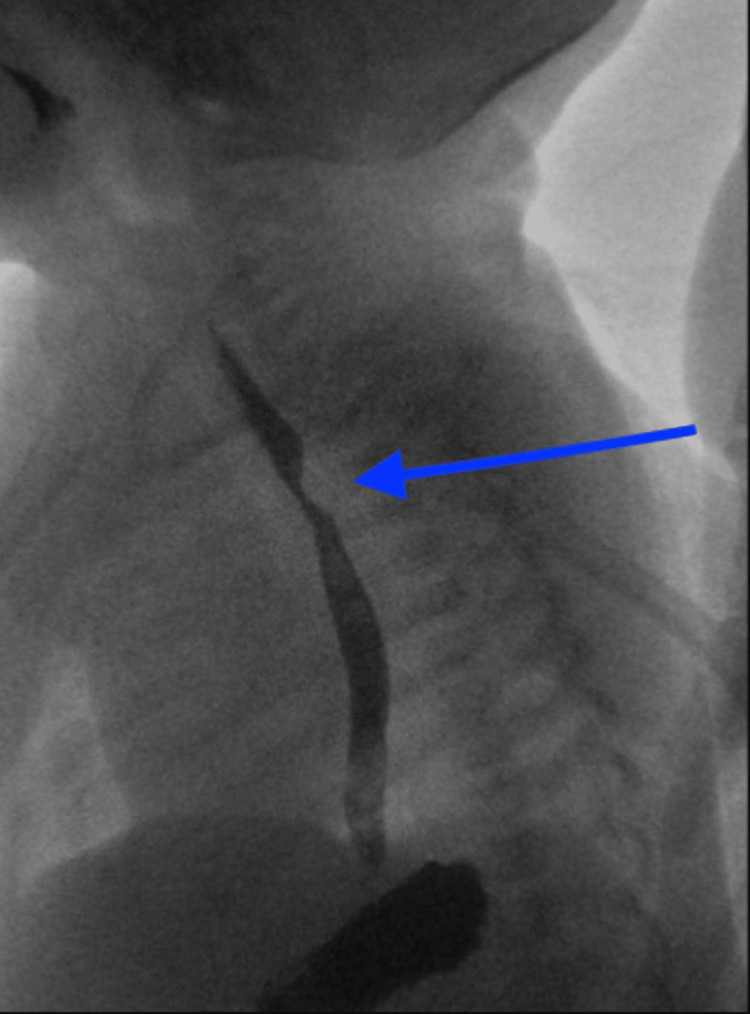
Fluoroscopy upper GI radiograph depicting a small posterior indentation of the upper esophagus, concerning for a vascular ring or sling GI: Gastrointestinal
